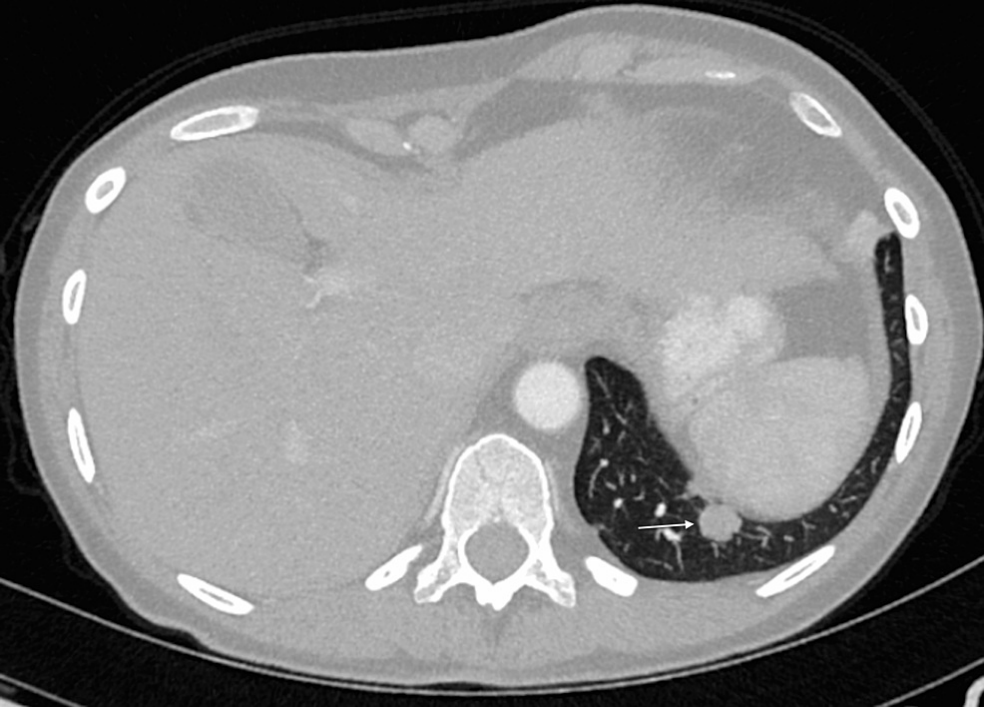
Axial CT demonstrating left lung base lesion in November 2019
